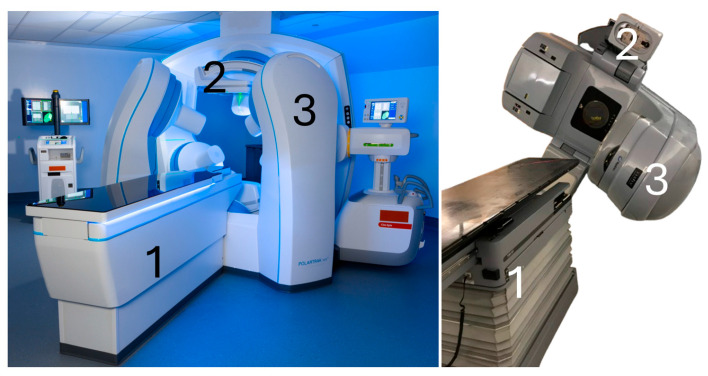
(Left) MTBT CuraDrone PolarTrak components (reproduced with permission from CuraDrone, Montréal, QC, Canada); (Right) IGRT Varian TrueBeam (reproduced under CC0 1.0). In both images, 1—robotic table, 2—CBCT, 3—radiation sources.
